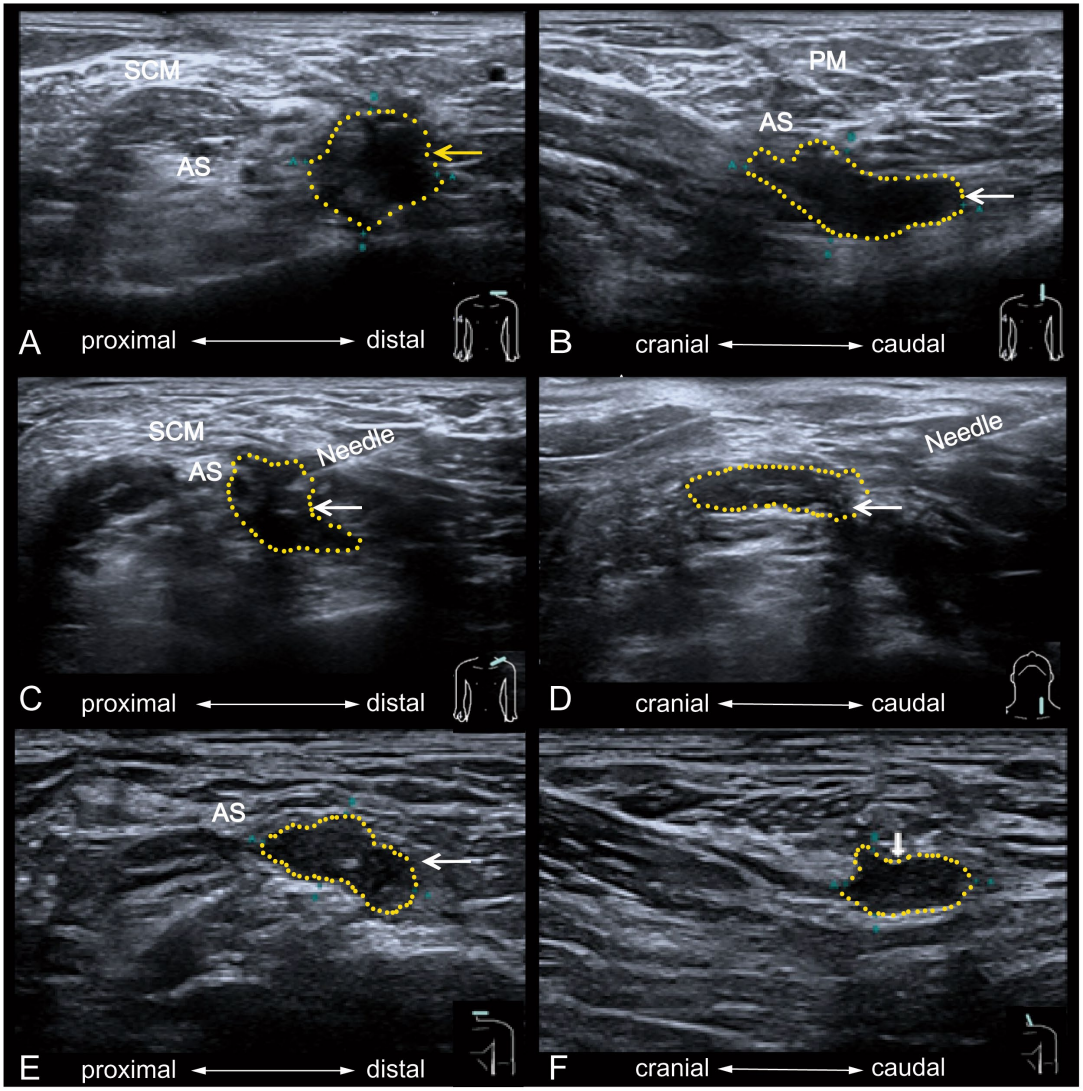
Ultrasound imaging before and after PRP injection treatment for brachial plexus neuroma. The left brachial plexus neuroma is seen in its short-axis (A) and long-axis (B) before the treatment. It shows a lack of a distinct envelope in the area of the left brachial plexus trunk lesion, the presence of irregularities, and structural disorganization of the nerve fibers. After ultrasound-guided injection in the brachial plexus neuroma is seen in short-axis (C) and longaxis (D). The short-axis (E) and long–axis (F) shows the image of the left brachial plexus neuroma after 6 months, and the neuroma decreases in tissue size. PM, platysma myoides. SCM, sternocleidomastoid muscle. AS, anterior scalene muscle. The yellow dashed area encircles the brachial plexus neuroma.
